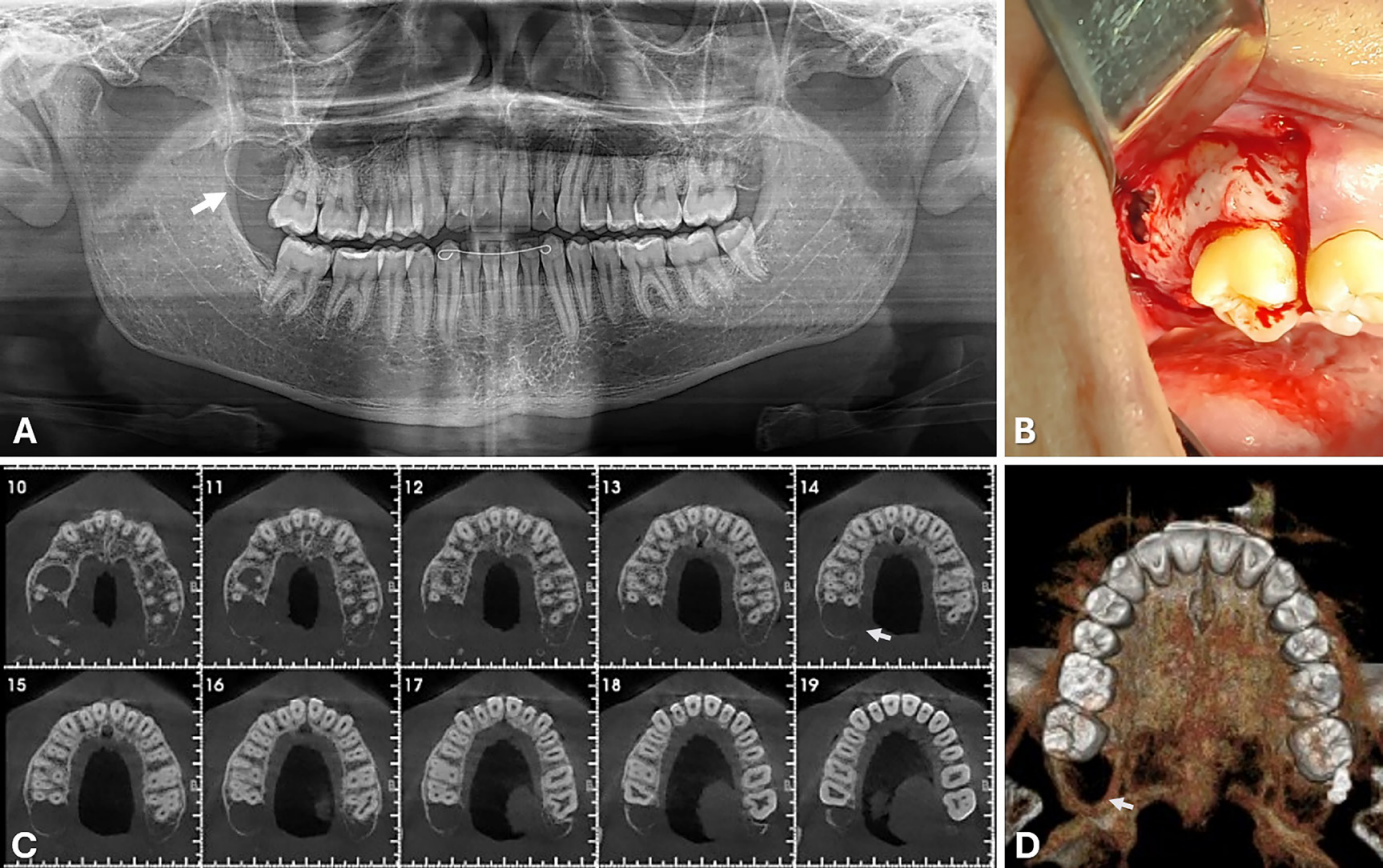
A: Clinical and imaginological features of the orthokeratinized odontogenic cyst in the posterior maxilla. Panoramic radiography revealed an unilocular, circular radiolucency surrounded by a radiopaque halo in the region of the left maxillary second molar (white arrow). B: Surgical access view demonstrating the precise localization of the cyst and a vestibular cortical rupture. C: Cone-beam computed tomography (CBCT), in axial reconstructions, confirmed the lesion’s proximity to the roots of the second molar, with no signs of root resorption and palatal and vestibular cortical thinning (white arrow). D: A three-dimensional reconstruction of the maxilla clearly delineated the well-defined lesion (D).
